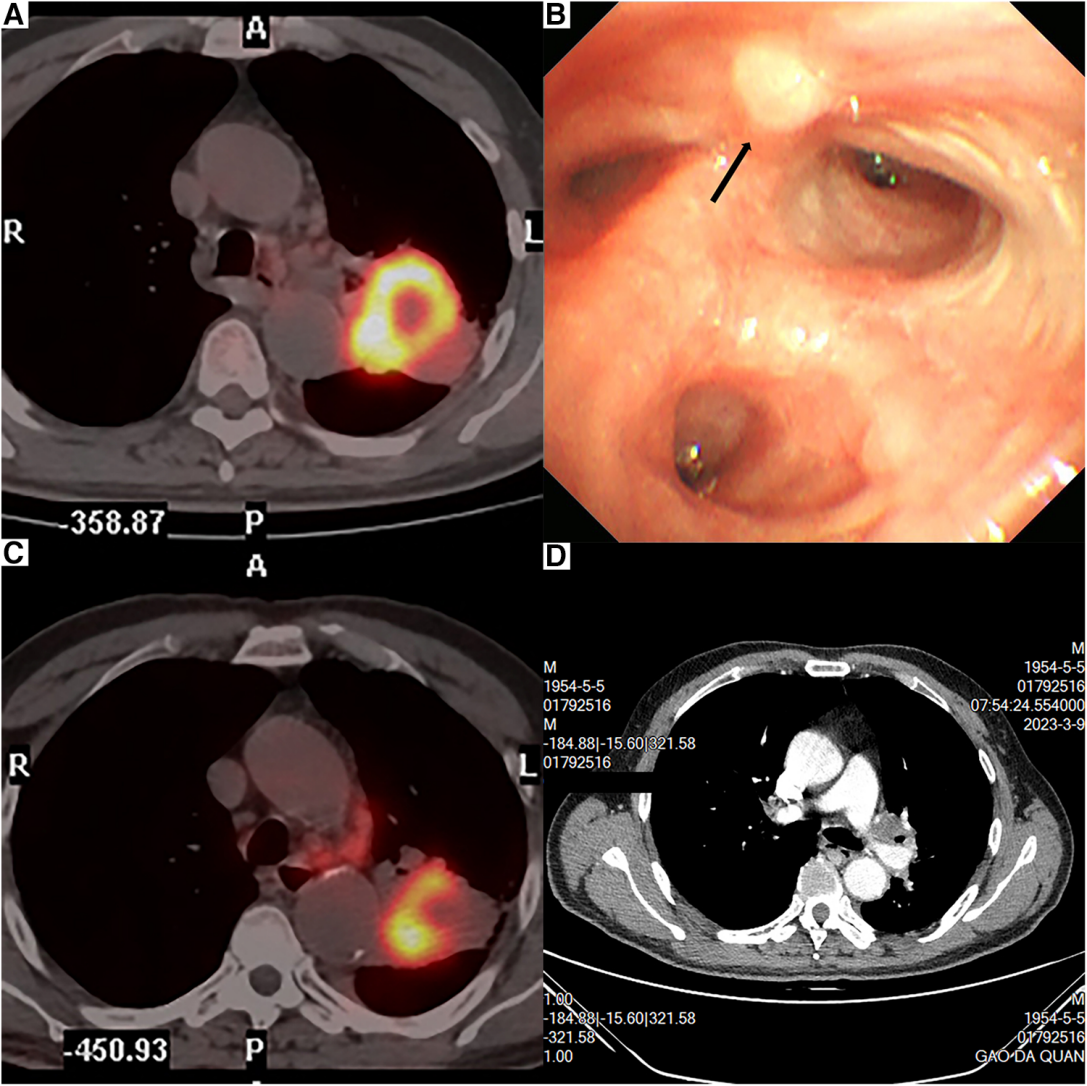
PET revealed a 61.7-mm × 43.9-mm tumor near the left hilum (A). PET examination after immunotherapy revealed that the tumor was 45 mm × 44 mm (B). Bronchoscopy result (C). Contrast-enhanced CT revealed that the tumor invaded the left upper bronchus and the left upper artery (D).
